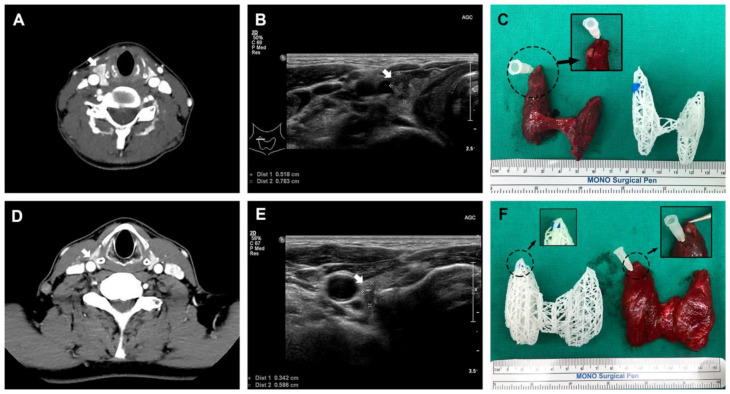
Two total thyroidectomized cases, which were clinically cT3bN1b. Each case had anterior (A–C) and posterior (D–F) external thyroidal extension into the upper pole on preoperative CT and ultrasonography, respectively (white arrow indicates thyroid cancer).
